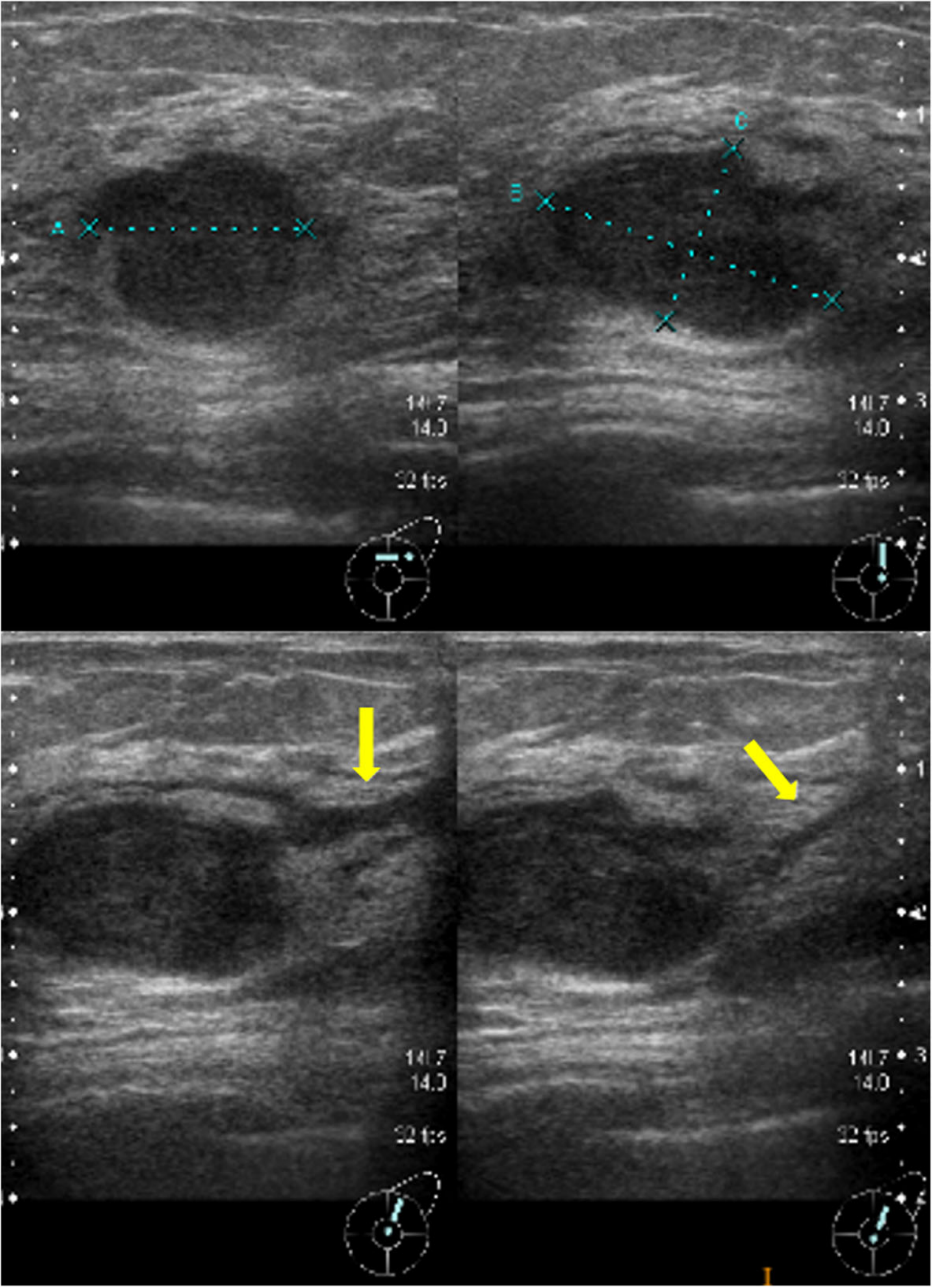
Ultrasonography shows a hypoechoic mass measuring 19 × 17 × 11 mm with an irregular margin, acoustic enhancement, and interruption of the posterior border of the mammary gland in the upper lateral quadrant of the left breast. It has connections with the surrounding mammary ducts (*arrows*)
